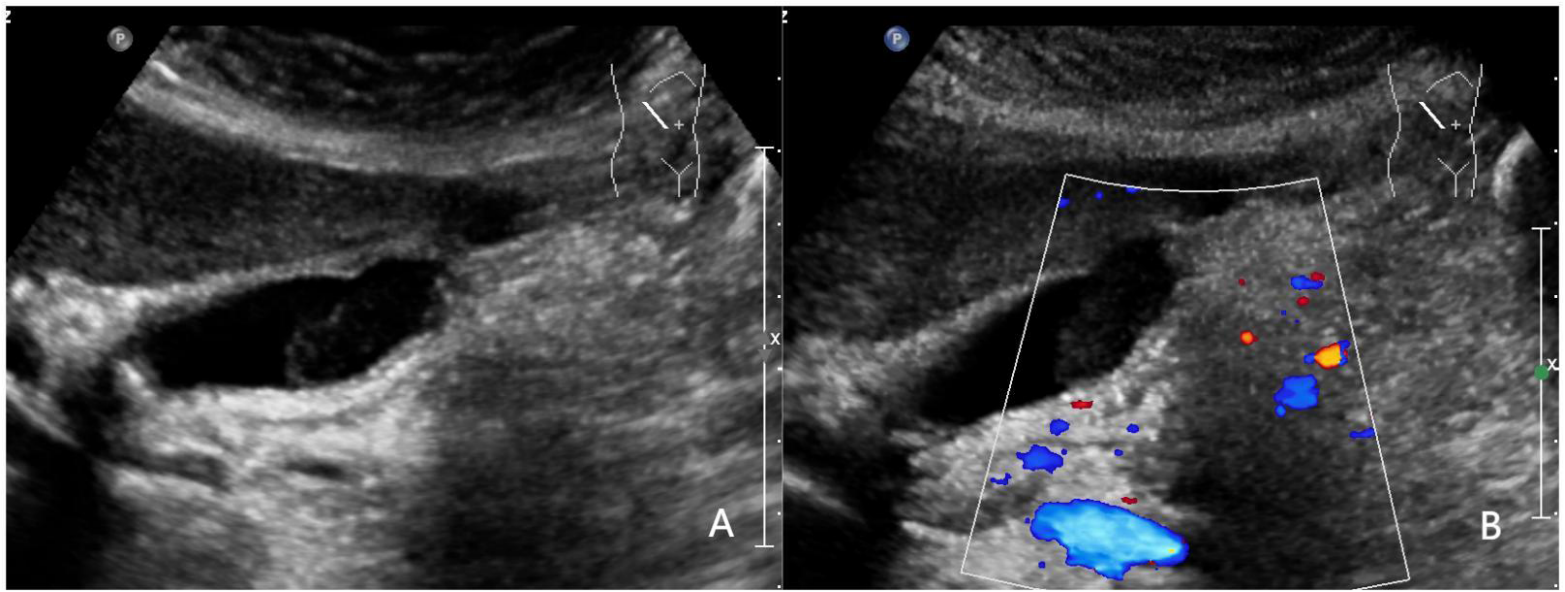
A 52-year-old woman with pathologically proven chronic cholecystitis. (A) Focal thickening of the base of the gallbladder, with no stratified appearance, and loss of interface with the liver.; (B) CDFI=0.Based on a consensus review of the sonographic findings, the lesion was categorized as GB-RADS 4, GB-RADS+CDFI 3.
